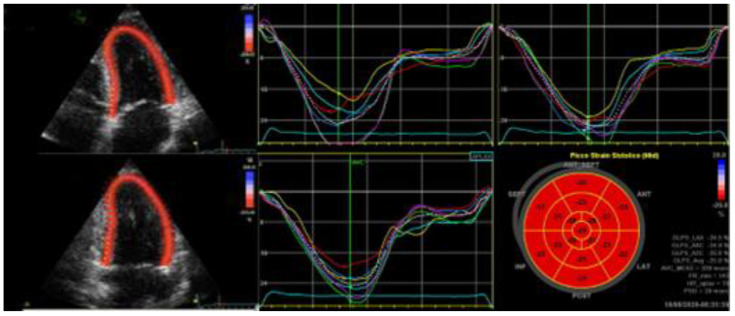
Two-dimensional speckle tracking: from recorded multiple heart beats during breath-hold, an apical four-chamber view, an apical two-chamber view, and an apical three-chamber view have been stored. Once the specific views have been named, it is required to trace the endocardial border in a still frame, setting the ROI. Finally, the evaluation of the deformation graphs and values may be calculated. The segmental peak systolic strains are represented in bullseye. ROI indicates region of interest.
